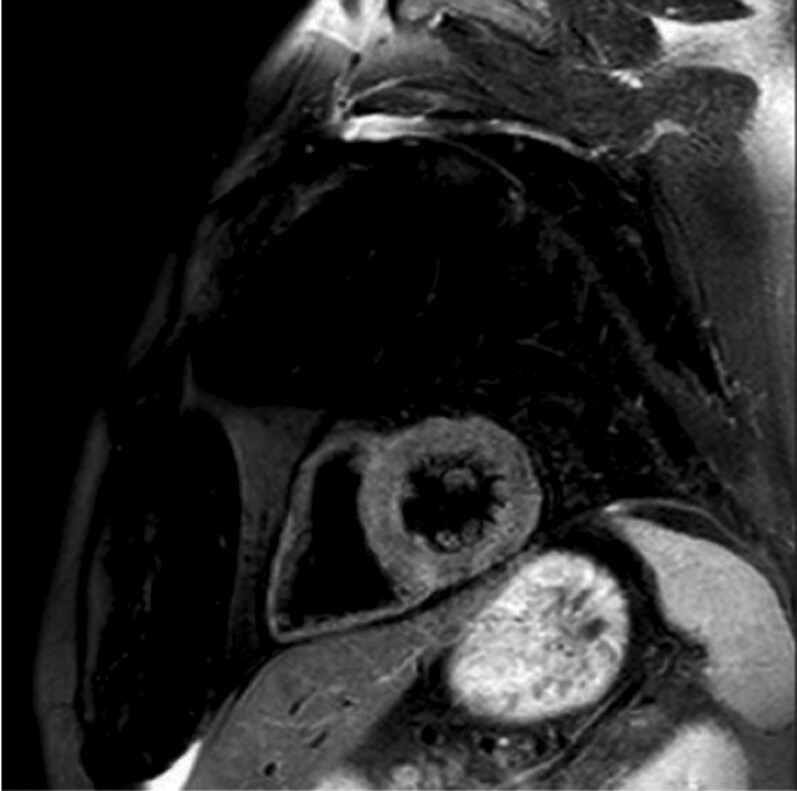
Cardiac magnetic resonance imaging. Cardiac MRI with T1 and T2 mapping demonstrating preserved biventricular function and no evidence of myocardial oedema, fibrosis, necrosis, or late gadolinium enhancement according to the updated Lake Louise Criteria, arguing against active myocarditis.
